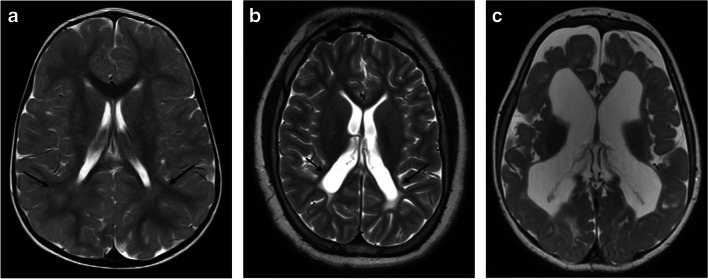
Grading of periventricular leukomalacia (PVL). Axial T2-weighted images of mild (a), moderate (b), and severe (c) PVL. a Mild PVL with subtle hyperintensity in the bilateral periventricular white matter adjacent to the atria (*arrows*) in a 19-month-old girl born at 31 weeks gestational age (GA). b Moderate PVL in a 14-year-old boy born at 27 weeks GA, characterized by abnormally high signal intensity in the periventricular white matter, as well as ventricular irregularity and mild dilatation (*arrows*). c Severe PVL associated with ventriculomegaly in an 11-month-old girl born at 27 weeks GA
